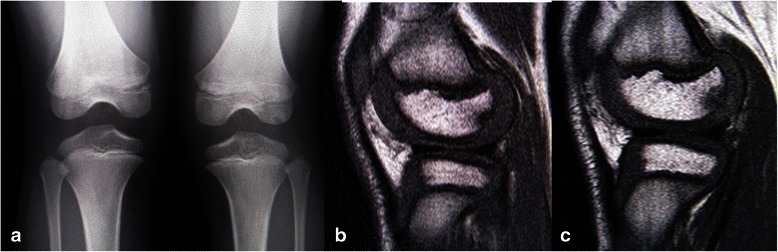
Image examinations at initial visit. a A posterior-anterior weight-bearing radiograph (Rosenberg’s view) at initial visit. An irregular radiolucent lesion with surrounding sclerosis is identified in the epiphyseal region. b, c T1-weighted sagittal magnetic resonance imaging images at first visit. An area of low signal intensity at the periphery of subchondral bone contour is present in the lateral femoral condyles of the patient’s right (b) and left (c) knees
